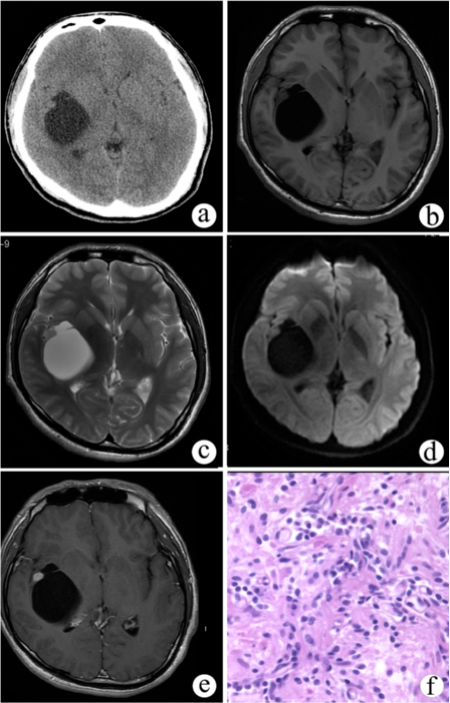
Cystic meningioangiomatosis with a cystic-mural nodule pattern. (a) CT scan showed a cystic-mural nodule lesion in the right insular lobe. (b-d) In non-enhanced MRI, the cystic content demonstrated similar signal intensity as cerebrospinal fluid (CSF), while the mural nodule demonstrated iso-signal intensity on T1WI, T2WI, and DWI. (e) On post-contrast MRI, the mural nodule demonstrated significant enhancement, while the cystic wall and content showed no enhancement. (f) Pathological examination showed perivascular spindle-cell proliferation.
